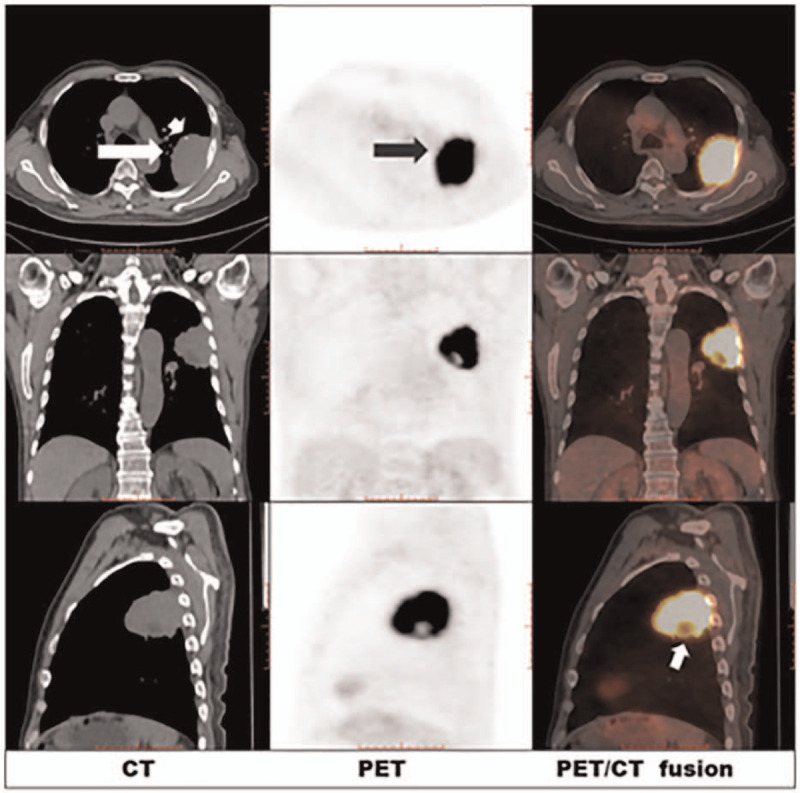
The CT images of the thoracic demonstrates a 5.7 × 7.9 cm lobulated solid mass with burr-like margins in the left upper lobe of lung (white arrow), with marginal punctate calcifications was noted (arrowhead). PET images reveal that the mass in left lung presenting intense FDG uptake (black arrow), with the SUV_max_ of 19.9. PET/CT fusion images the short white arrow indicate a local decreased FDG radioactivity in the lower margin of the lesion. SUV_max_ = the maximum of standard uptake value.
